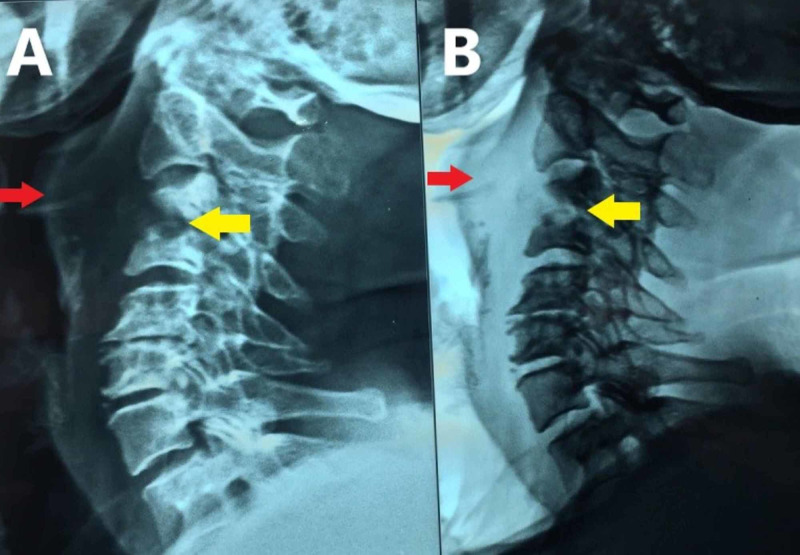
Lateral-view plain radiograph (A) and digital subtraction radiograph (B) revealing soft tissue mass (red arrows) in the prevertebral area displacing the airway anteriorly, and focal destruction (yellow arrows) of C3 and C4 vertebrae
